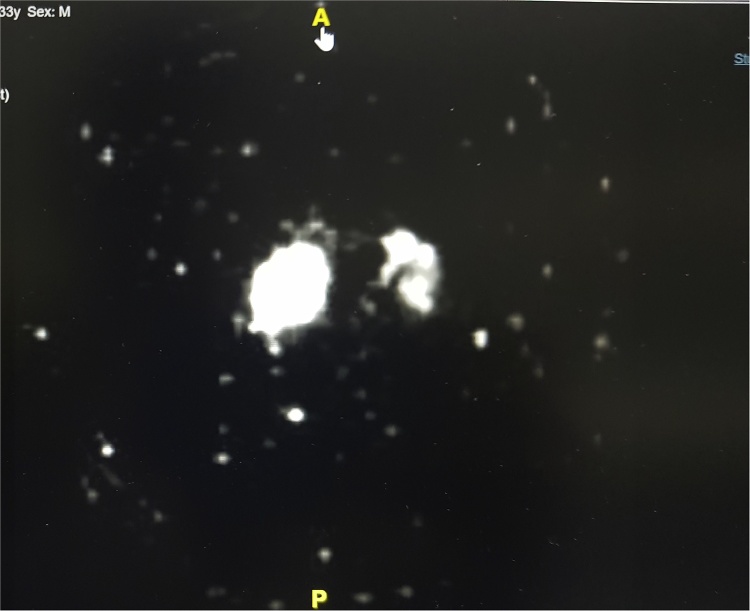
18 FDG PET-CT (Non-contrast) showing metabolically active left internal and external iliac lymph nodes.
